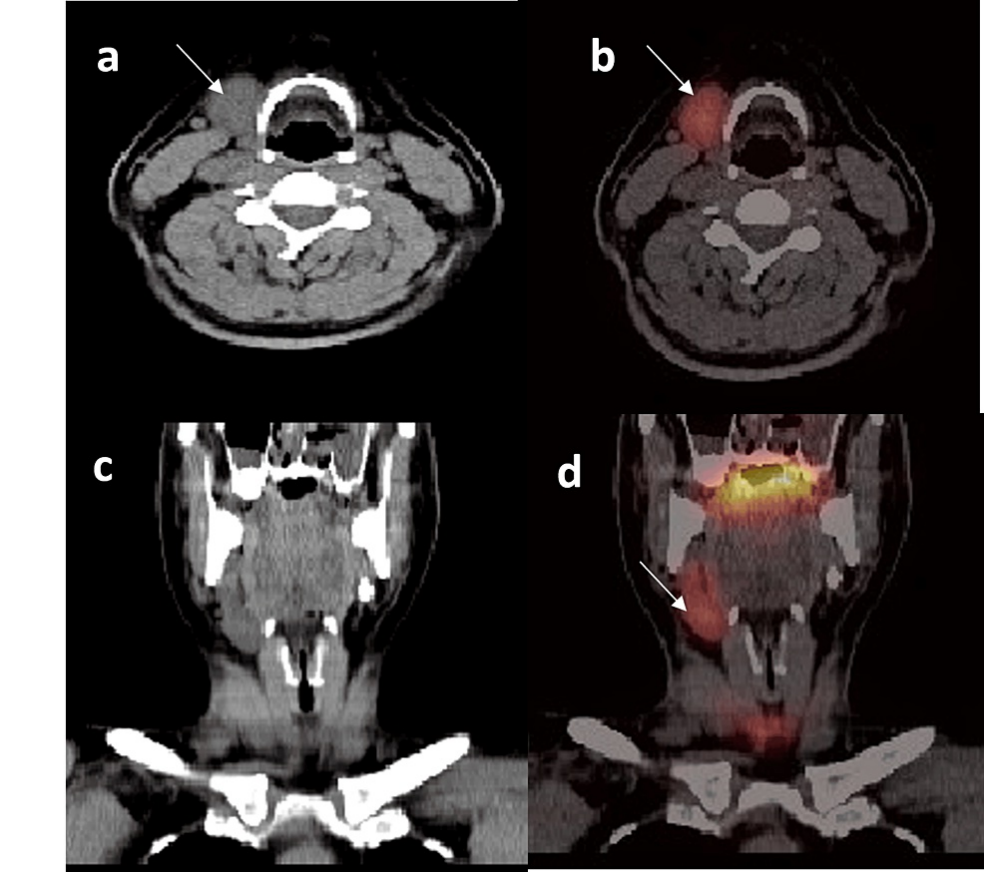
SPECT/CT images showing soft tissue structure (white arrow) on the right side of the neck with similar radiotracer uptake to the thyroid gland and absent submandibular gland on the left side. a) Axial unenhanced CT scan of the neck, b) axial fused SPECT/CT scan of the neck, c) coronal unenhanced CT scan of the neck, and d) coronal fused SPECT/CT scan of the neck.
